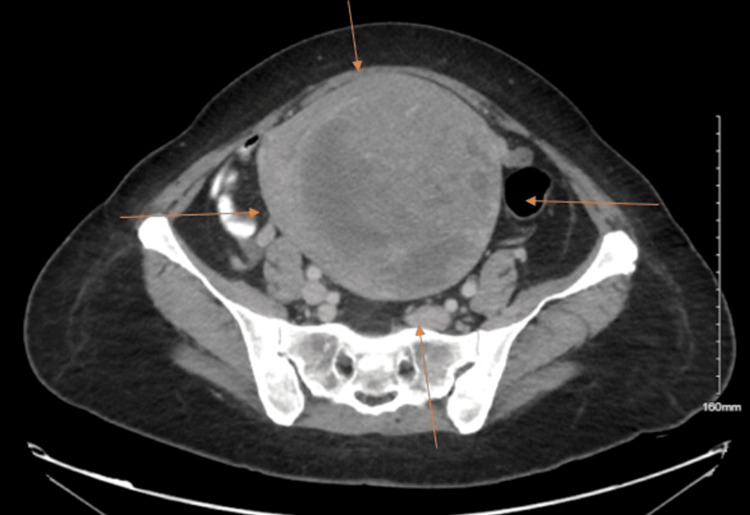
Computed tomography of abdomen and pelvis revealing a large tumor Arrows point toward the mass
